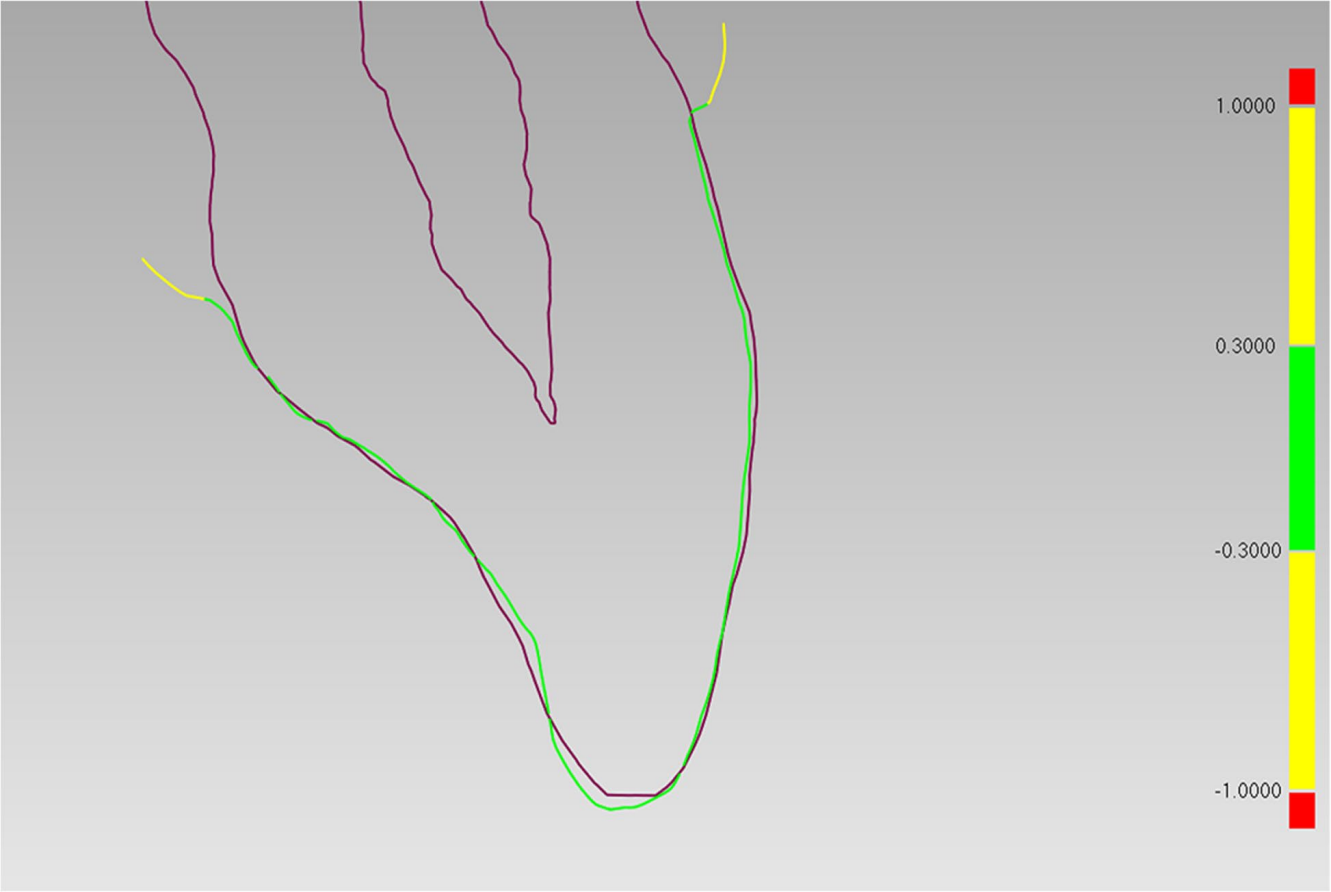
On the cross-sectional plane determined by the long axis and the gingival zenith of the adjacent tooth, the purple line segments represented the contour of the adjacent teeth obtained from CBCT scans. While the other line represented the contour of the crown obtained from IOS. Since the 2D deviation of corresponding points all fell within ± 0.3 mm, the crown contour was in green
